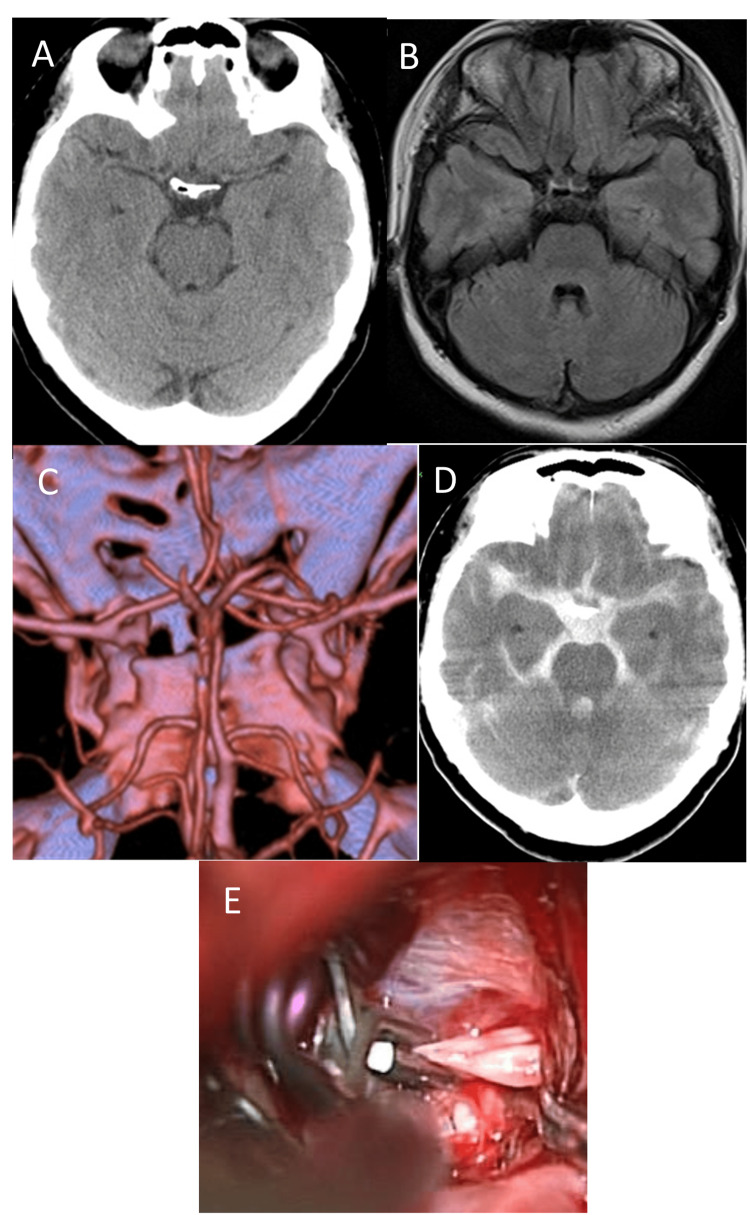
Case 3 CT (3A) and FLAIR-MRI (3B) scans at the initial examination. No SAH was detected. CT angiography (CTA) revealed the inferolateral-directed ICA-PcomA aneurysm with bleb (3C). Shortly after the CTA, the patient became comatose, and a CT scan taken at that time (3D) showed rebleeding (Fisher 3). Intraoperative findings (3E) revealed that the aneurysm firmly adhered at a site behind the anterior clinoid ligament.
